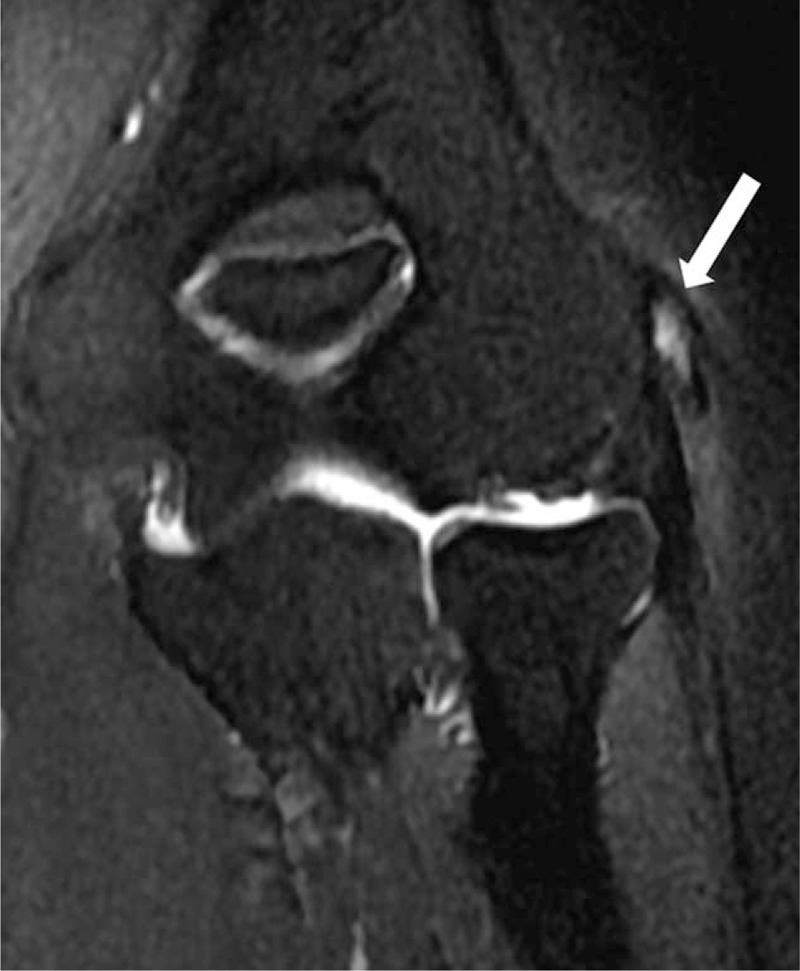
54-year-old man with left elbow pain ∼1.6 years (Tendinopathy score = 2; PRTEE score = 57). Coronal T2-weighted fat-suppressed MR image shows moderate focal increased signal in tendon (white arrow). PRTEE = Patient-Rated Tennis Elbow Evaluation.
